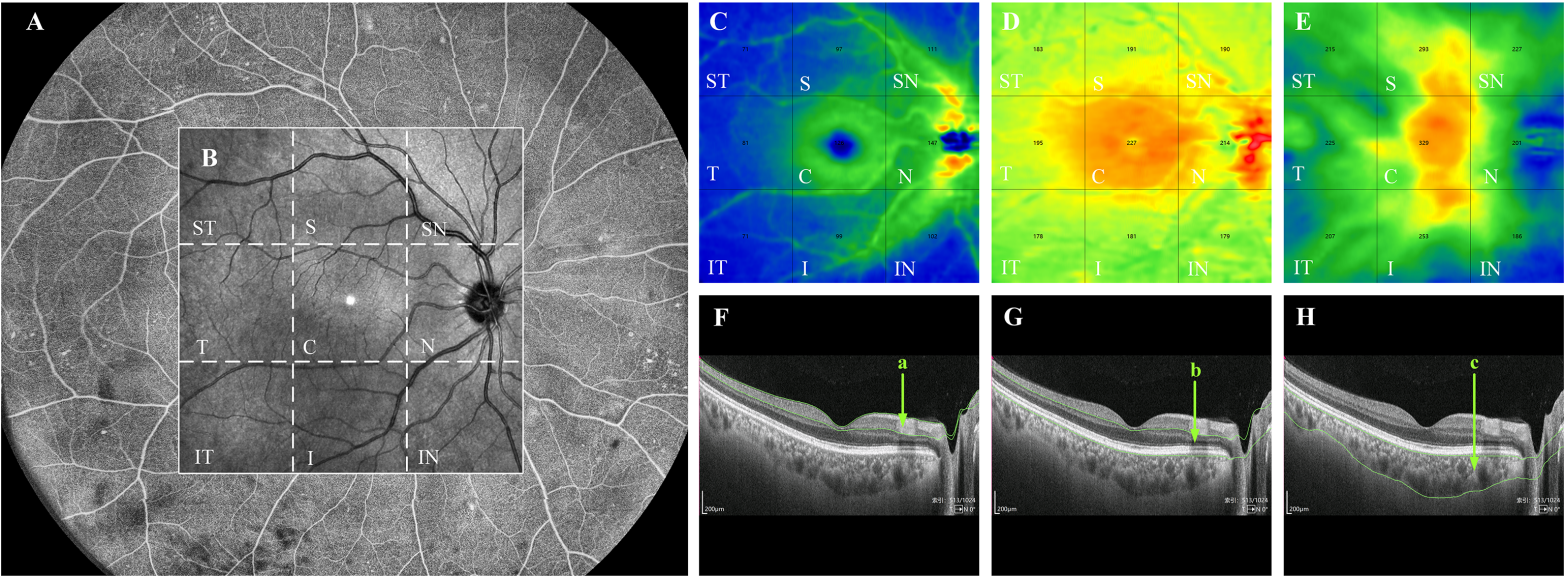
Quantification of thickness in each layer of the fundus by WSS-OCTA. WSS-OCTA can scan fundus images in the range of 24mm×20mm (A). In this study, fundus image data in the range of 12mm×12mm were selected for quantitative thickness analysis (B). Each cross-sectional image obtained by WSS-OCTA scanning was automatically sectioned by the built-in software into three thickness topographic maps, namely, IRL, ORL and choroid (C–E), each image is divided by the built-in software into nine regions, namely, ST, S, SN, T, C, N, IT, I, and IN regions. The thickness of each region in each layer is quantified. (F–H) are the imaging tomography at the level of the macular fovea, where the distance between the green lines in (a–c) indicate the IRT, ORT, and CT, respectively. IRL, inner retinal layer; ORL outer retinal layer; ST, supratemporal; T, temporal; IT, inferotemporal; S, superior; C, central macular area; I, inferior; SN, supranasal; N, nasal; IN, inferonasal; IRT, inner retinal thickness; ORT, outer retinal thickness; CT, choroidal thickness.
